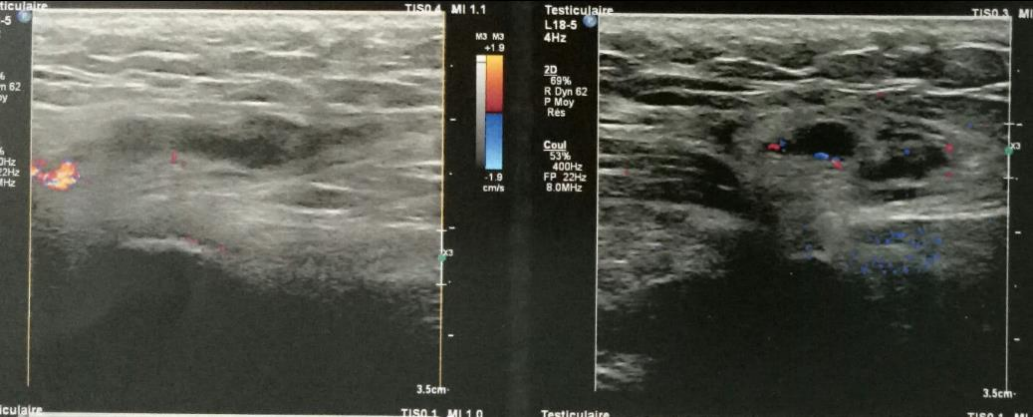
color Doppler signal was not presented inside the hypoechoic structure
